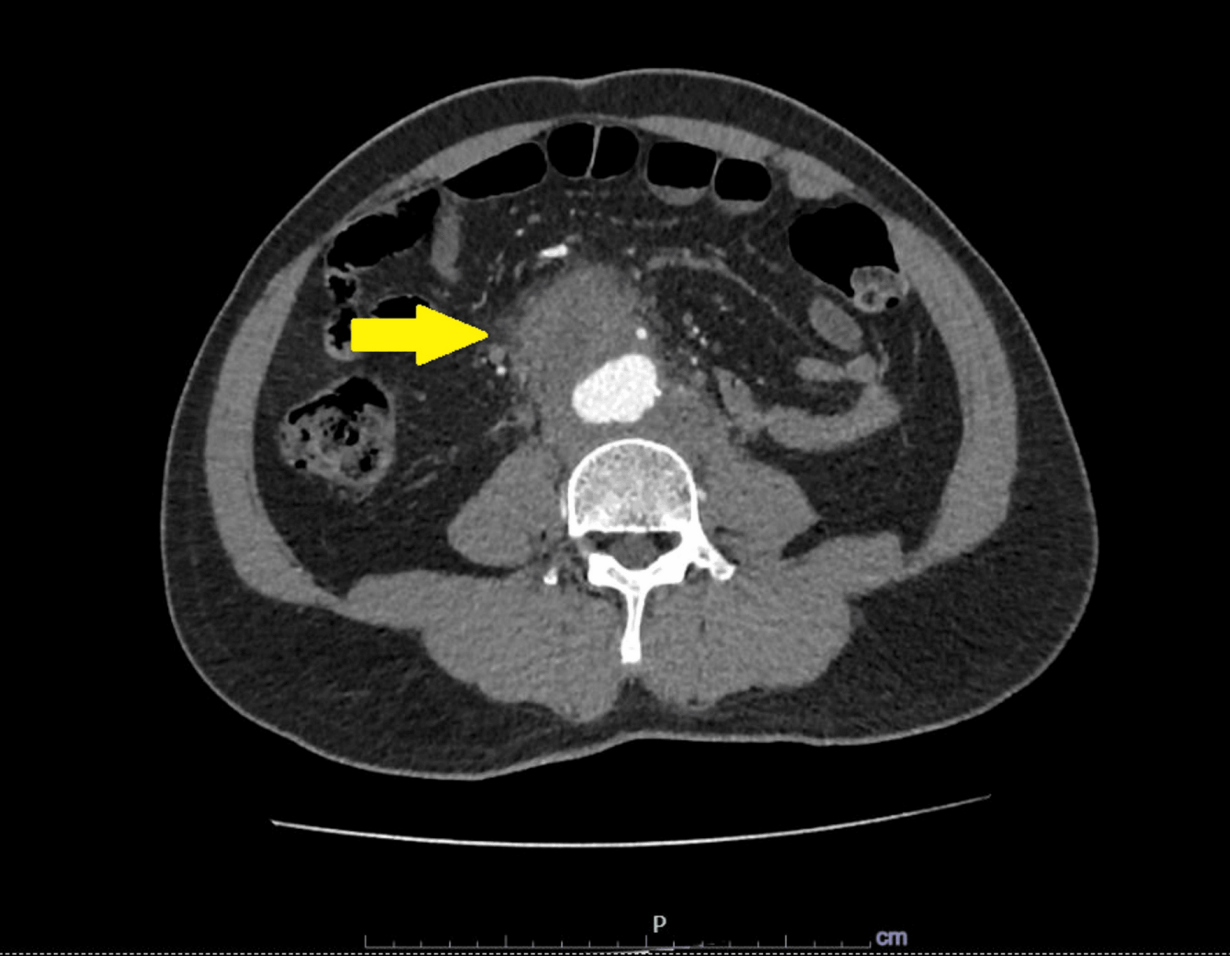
Axial view of the CT scan of the abdomen showing the presence of surrounding perianeurysmal hematoma extending anterolaterally into the right retroperitoneal region, measuring approximately 5.6 x 4.5 x 6.8 cm
